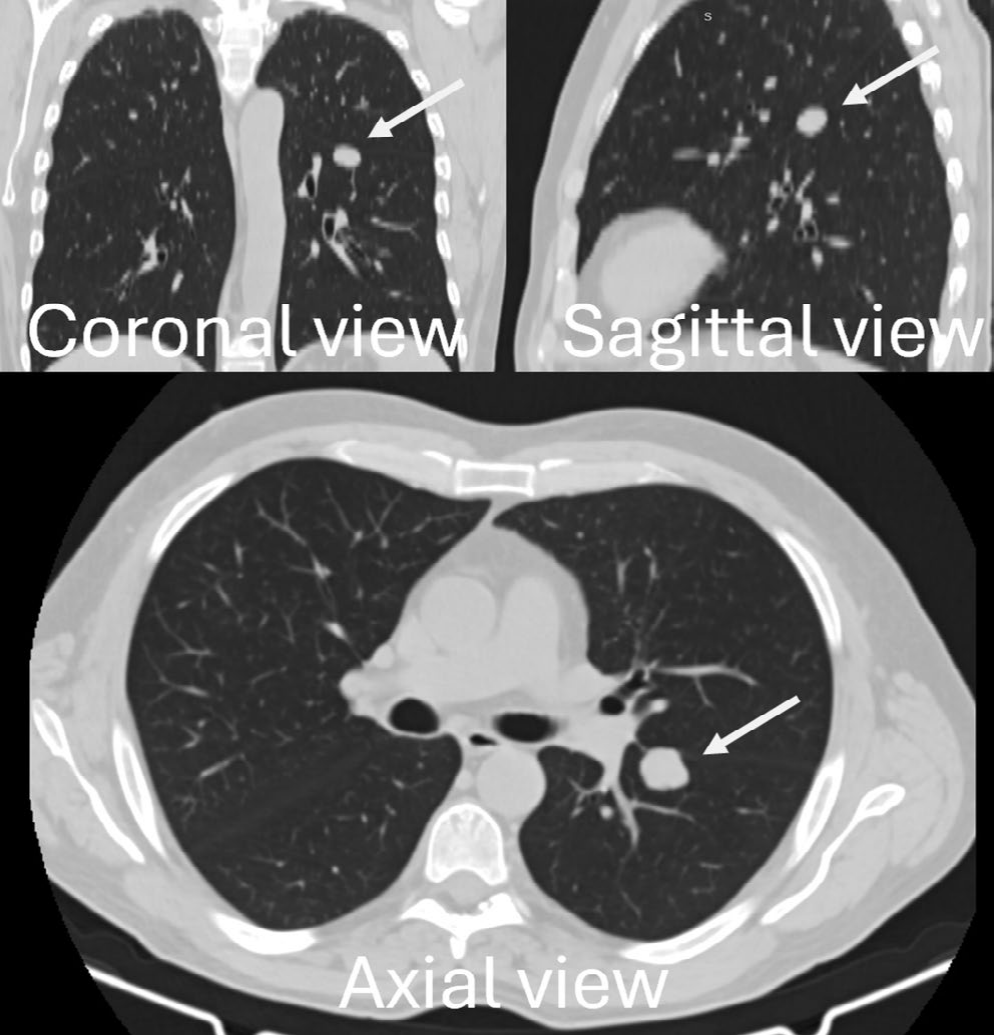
Multiplanar reconstruction of chest computed tomography scan showed a 20 mm nodule (white arrow) localized within superior segment of the left lower lobe.
